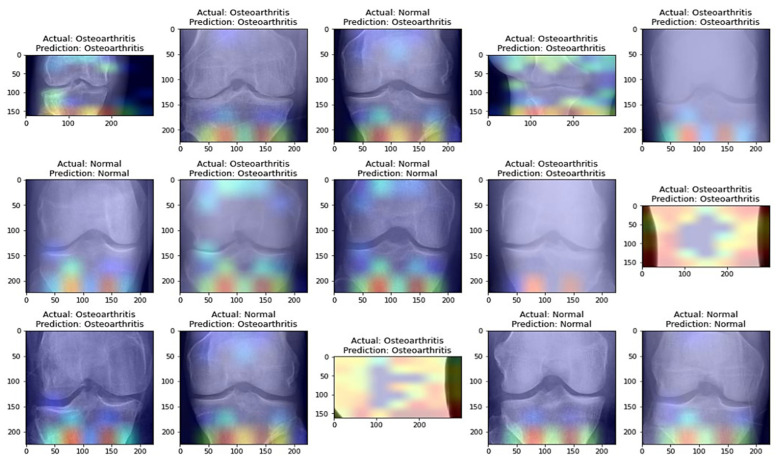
Predictions obtained with the most accurate model on randomly selected X-ray images from the dataset.
